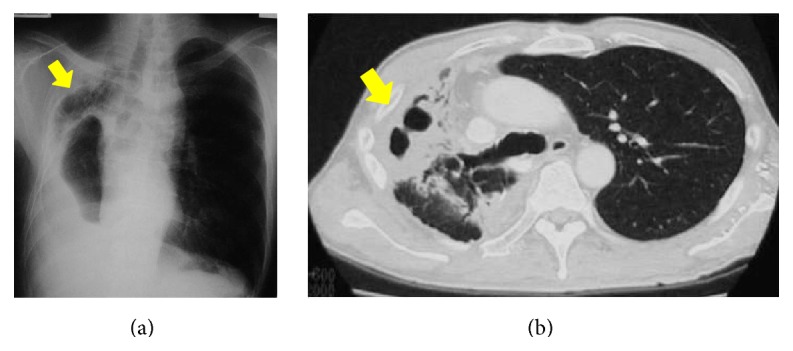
Chest X-ray (a) and chest contrast tomography scan (b) in Case 2. This patient had repeated infections and severe destroyed lung in which almost all the existing lung structures were destroyed.
